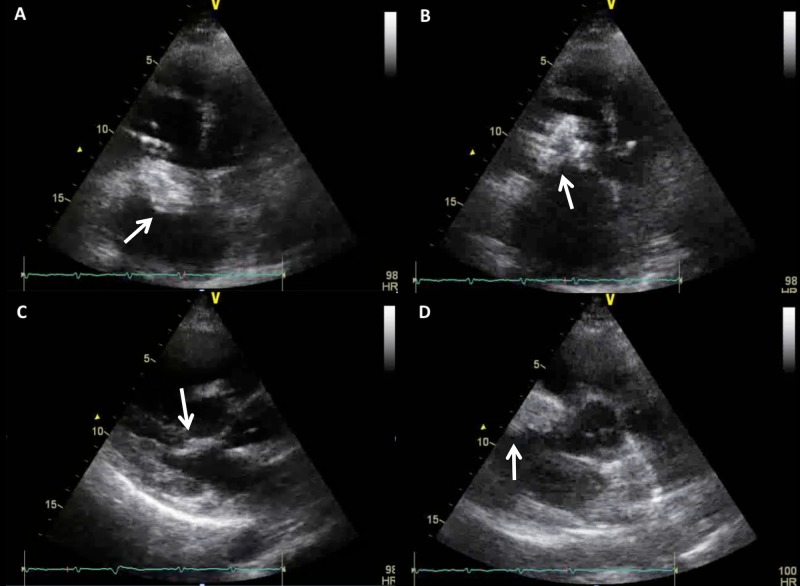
Findings on transthoracic echocardiography. (A-C) A dense echogenic structure, measuring 4.7 cm x 2.7 cm, with areas of calcification was seen attached to the anterior leaflet of the tricuspid valve (TV) and protruding into the right ventricle. (D) No right ventricular outflow tract or pulmonary artery involvement was noted.
